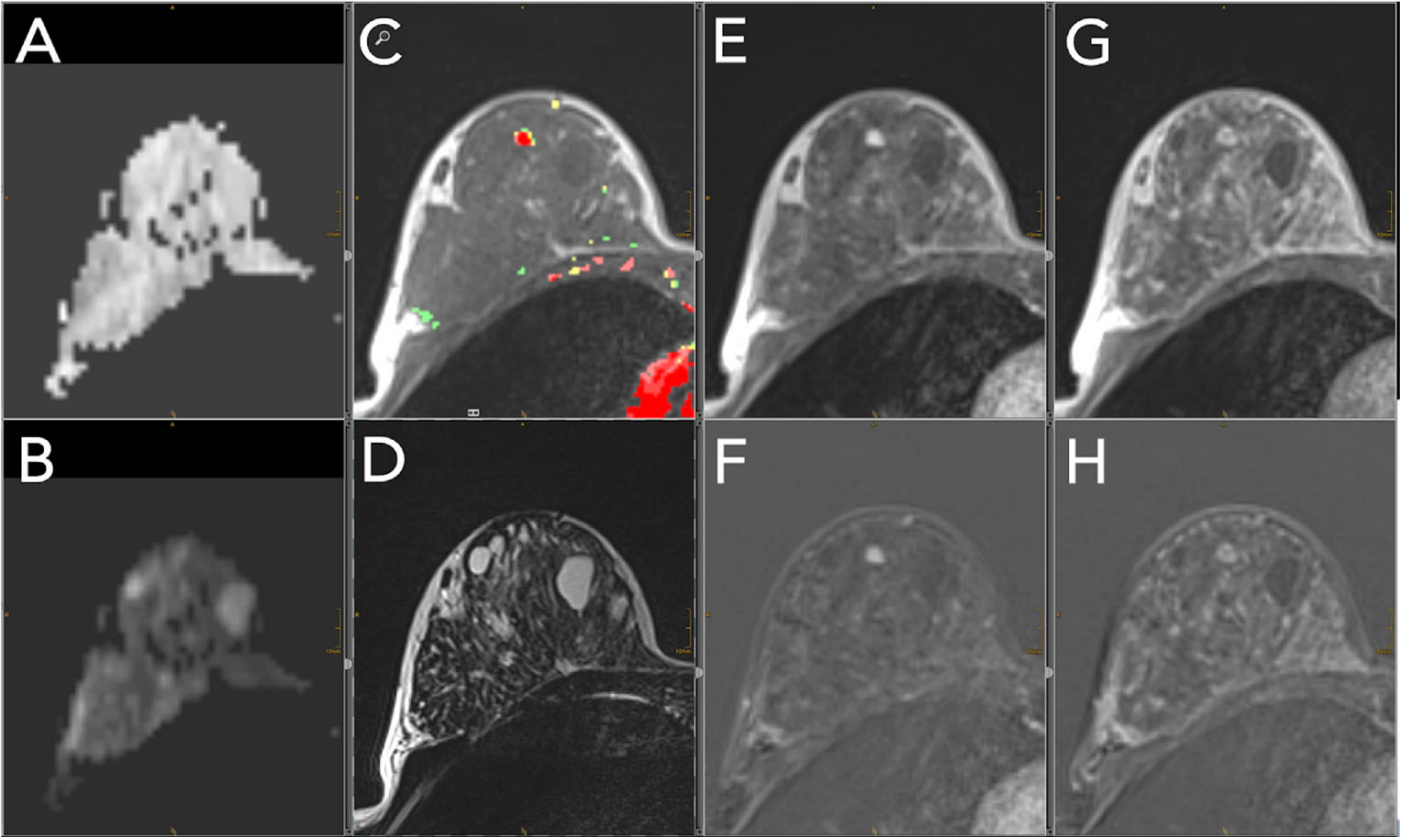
Standardised hanging protocol/reading layout for a two-screen solution. Standardised hanging protocols decrease the reading time and increase diagnostic confidence while reducing potential sources of errors. All series should be linked, allowing simultaneous scrolling and zooming. This example shows a 44-year-old high-risk patient. A: ADC map; B: high b-value DWI image; C: pre-contrast T1w (here with superimposed colour-coded enhancement map indicating dominant wash-out, coded in red); D: T2w-TSE; E/F: early contrast-enhanced scan with corresponding subtraction; G/H: delayed contrast-enhanced scan with corresponding subtraction within the dynamic series (E–H); the same windows and centre setting should be used. This step should be performed for subtracted and non-subtracted images separately. An incidental lesion of 5 mm in size is present in the right breast, showing homogeneous enhancement (E, F), followed by early and central wash-out (C, G, H). Intermediate T2w-signal [D, no restricted diffusivity (A, B)]. The lesion corresponds to a Kaiser score of 4 (see Fig. 6); MRI-guided biopsy revealed a benign adenosis B2
